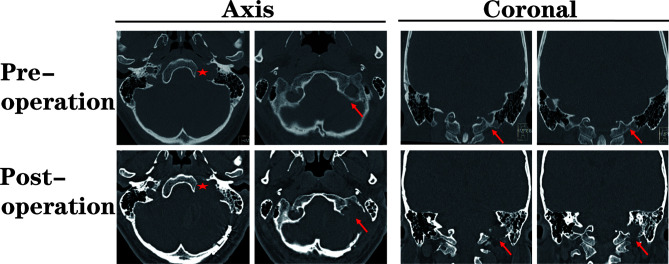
The pre- and post-operational high-resolution computed tomography (HRCT) indicated the extent of paracondylar bone removal. During the suboccipital paracondylar-lateral cervical approach, the jugular process was precisely removed while the condyle was kept intact. Red star: enlarged jugular foramen; Red arrow: the extent of removed bone mass.
